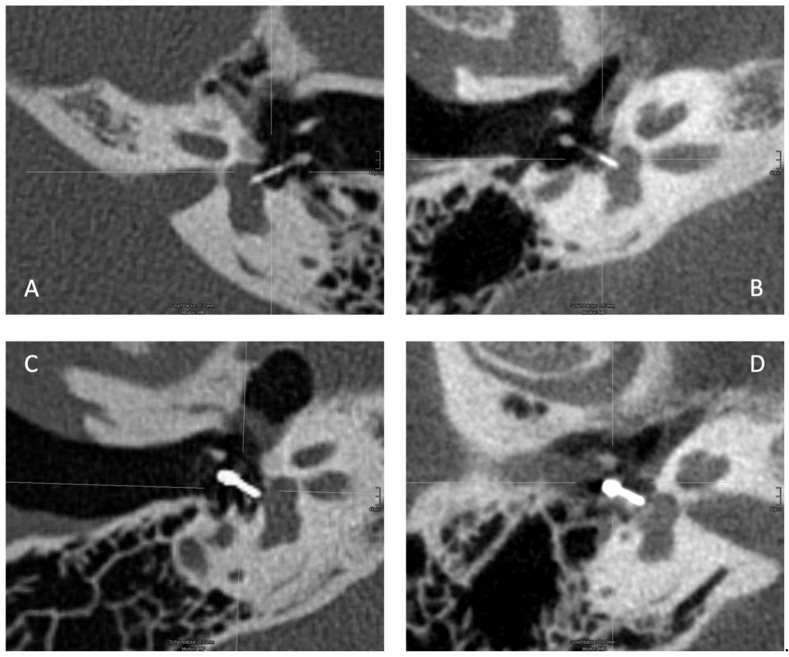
(A) Titanium piston on CT. (B) Titanium piston on CBCT. (C) Platinum–fluoroplastic piston on CT. (D) Platinum–fluoroplastic piston on CBCT. (CT—computed tomography, CBCT—cone beam computed tomography).
